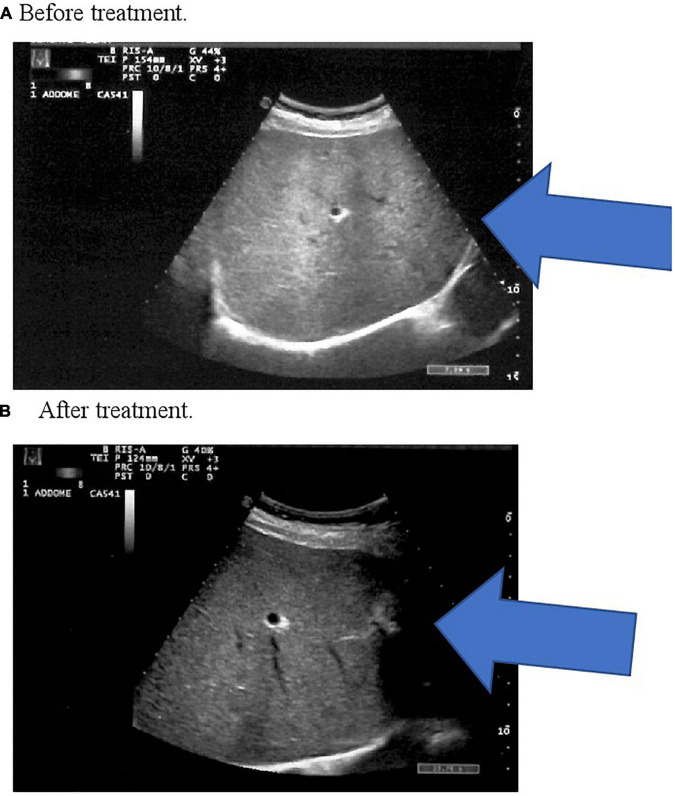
(A) Before treatment. (B) After treatment. In panel (A) there is an increased echogenicity of the liver, a classic sonographic finding of hepatic steatosis. In panel (B) ultrasound image shows normal echogenicity of the liver parenchyma.
